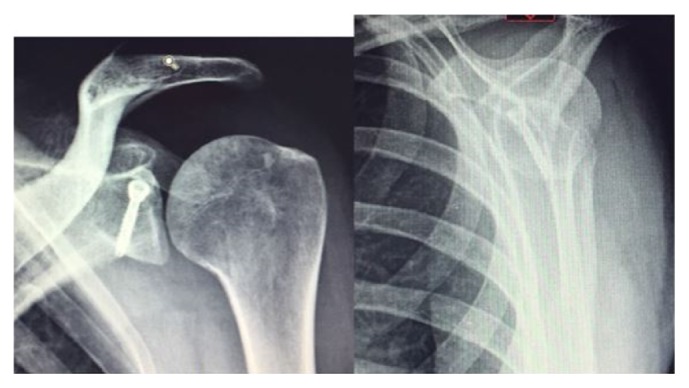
AP and lateral X-ray at 6-month follow-up.
